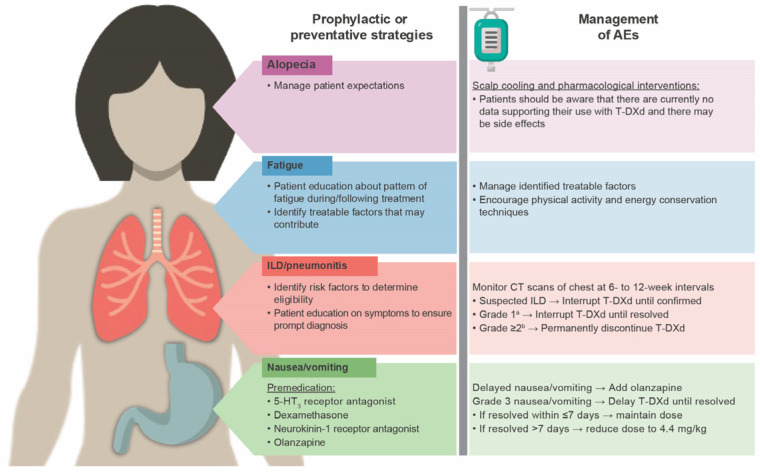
Monitoring and management of T-DXd–related AEs [5,119,121,124,132]. 5-HT_3_, serotonin receptor; AE, adverse event; CT, computed tomography; ILD, interstitial lung disease; T-DXd, trastuzumab deruxtecan. ^a^ Asymptomatic, radiographic findings only. ^b^ Grade 2: Symptomatic, mild respiratory symptoms that do not interfere with daily living. Grade 3: Symptomatic, interfering with daily living, possibly needing oxygen therapy. Grade 4: Severe, disabling symptoms leading to hospitalization and ventilatory support. Grade 5: Death.
